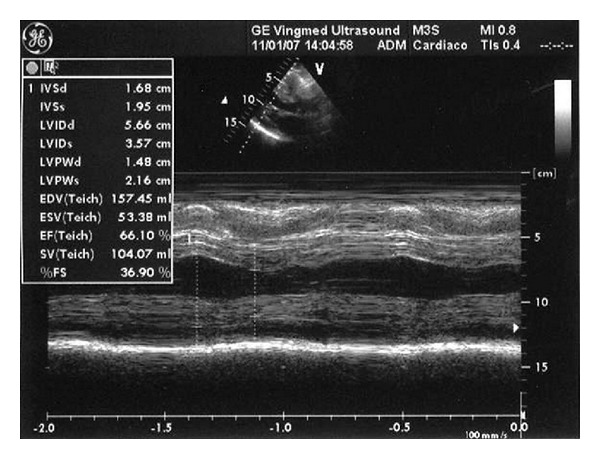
Case 2; echocardiography after the melphalan/dexamethasone cycles.
